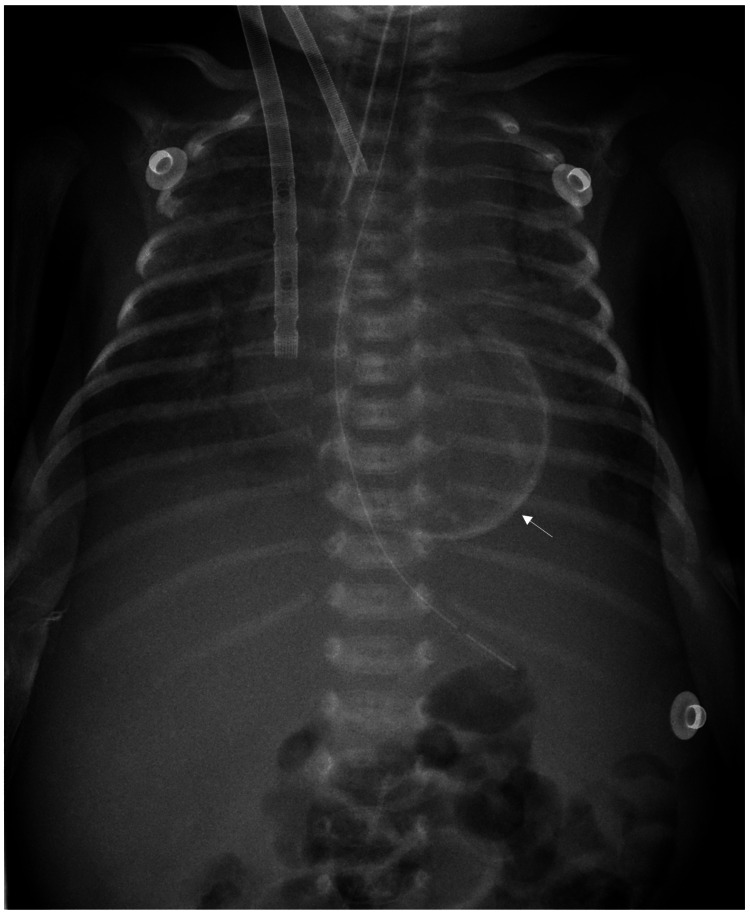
Chest radiograph showing myocardial calcification (arrow), visible as a smooth, eggshell-like curvilinear outline encircling the cardiac silhouette. The presence of this radiographic feature indicates advanced myocardial injury and correlates with poor prognosis
